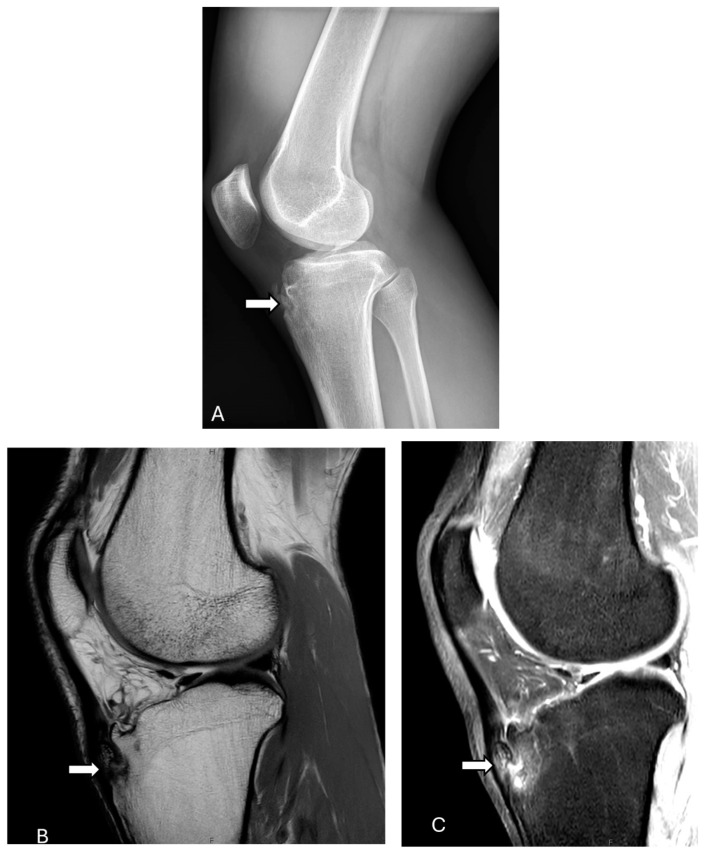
Osgood–Schlatter disease. Lateral knee X-ray (A), sagittal T1-weighted (B), and PD fat-suppressed (C) images show well-corticated ossific densities adjacent to the tibial tuberosity (arrows). These ossifications follow the signal intensity of bone on MR images, likely sequela of Osgood–Schlatter disease. The images were obtained from the University of Washington Medical Imaging database with the required permissions.
